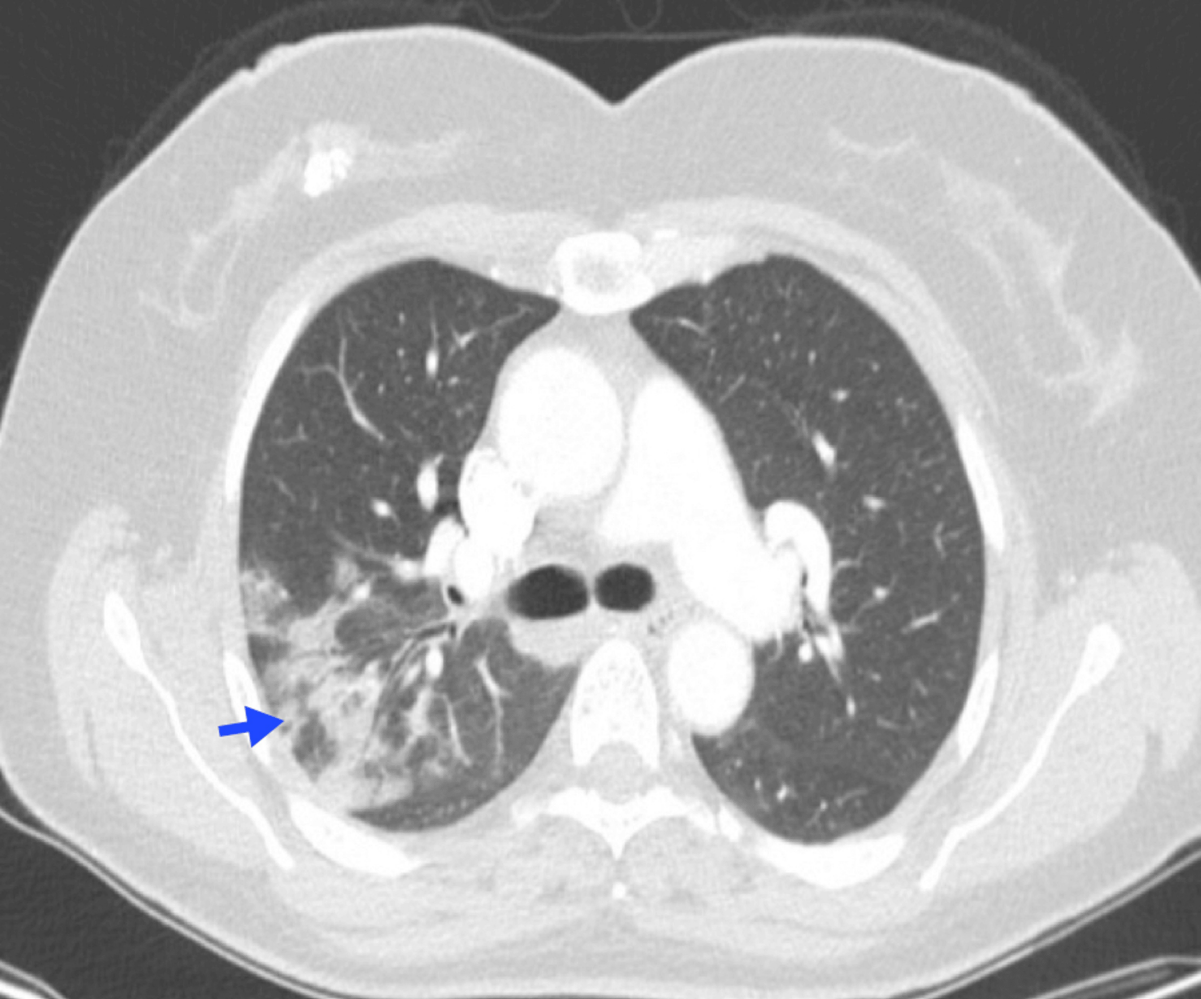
Cryptogenic organizing pneumonia A 53-year-old female patient with splenic marginal zone lymphoma. Chest CT reveals subpleural and peribronchial consolidations, along with multifocal ground-glass opacities in the posterior segment of the upper right lobe and lateral segment of the middle lobe (blue arrow). Image courtesy: Pulmonology Service of the Internal Medicine Department, University of Antioquia. Consent for open access publication was obtained from the patient.
